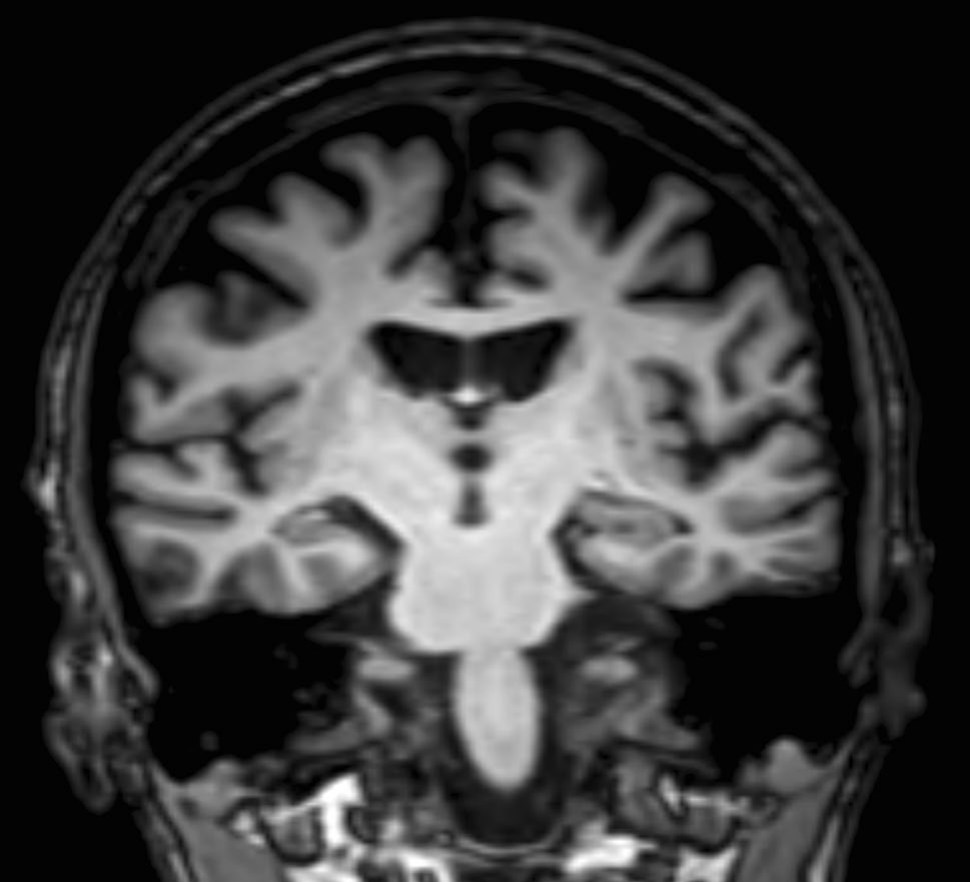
Mild atrophy on coronal T1-weighted MR image of the patient at the age of 56 years
